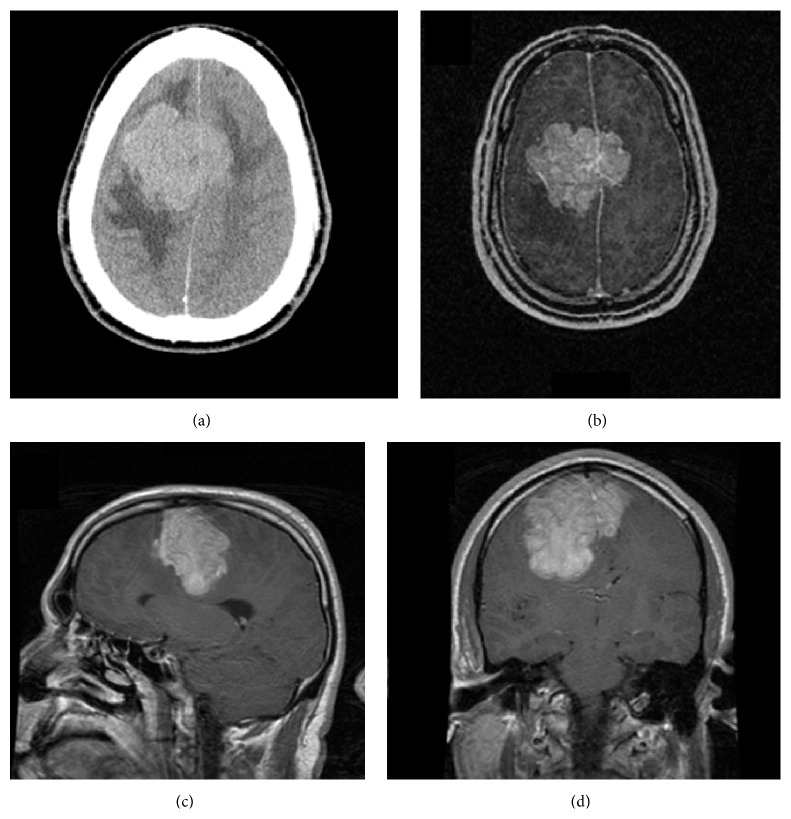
Axial, noncontrast CT scan (a) demonstrating a hyperdense, bifrontal, parasagittal lesion with surrounding vasogenic edema. T1 weighted, postcontrast MRI images showing (b) axial, (c) sagittal, and (d) coronal views of the mass.
